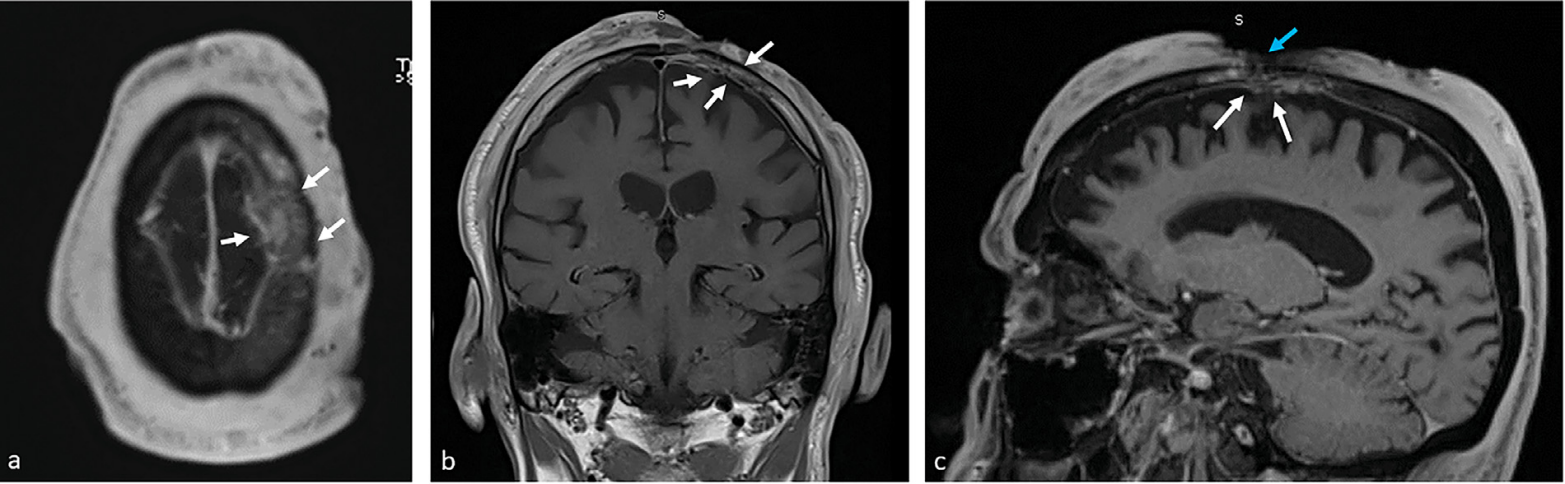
MRI head of the patient with cutaneous angiosarcoma of the scalp after local excision of the lesion. Axial (A) and coronal (B) post gadolinium contrast T1-weighted images, and sagittal (C) MPRAGE image demonstrate residual part of the lesion that is infiltrating the dura and causing dural enhancement (white arrows), and infiltrating the left frontal and parietal bone (blue arrow). Findings indicate local invasion of the tumor (Color version of the figure is available online.).
